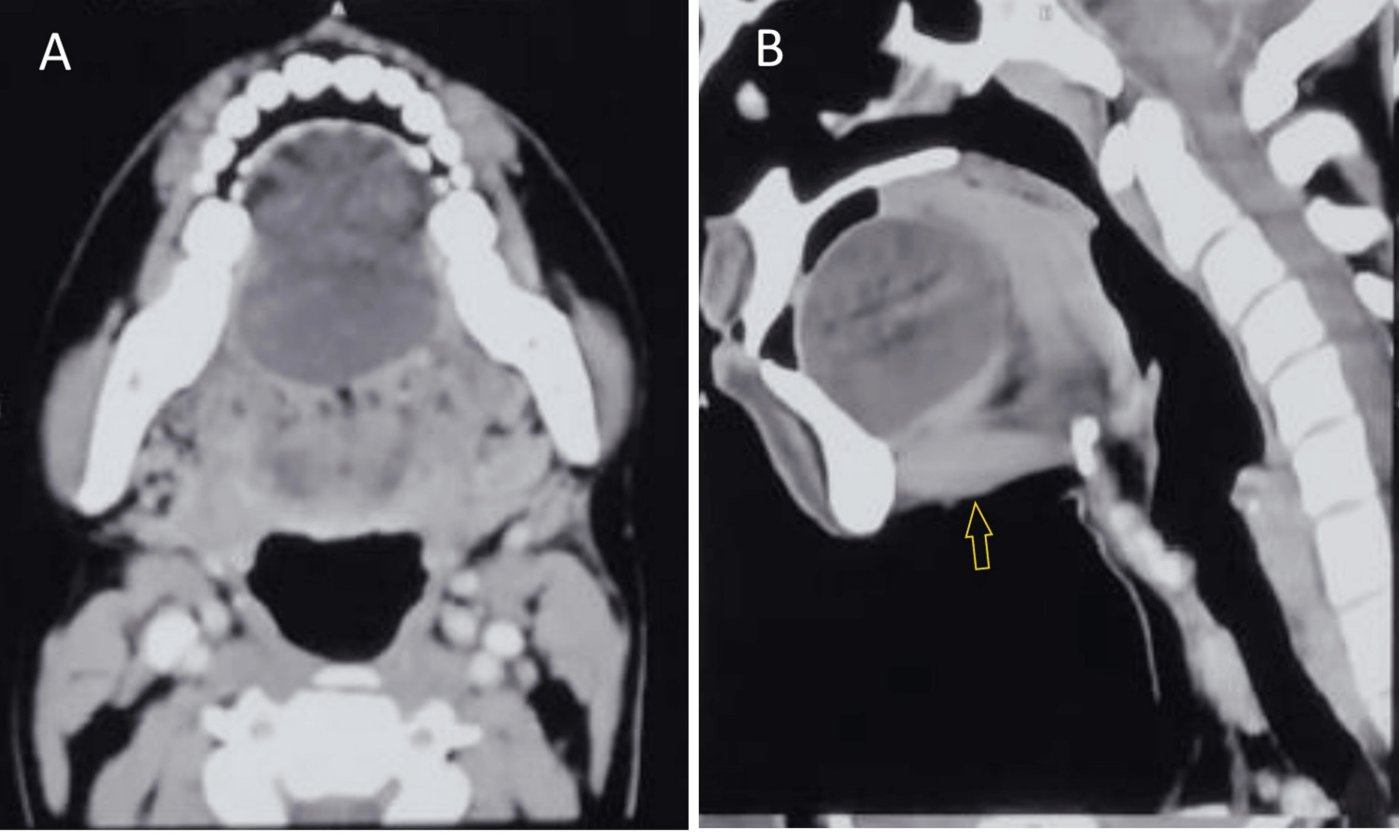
Axial (a) and sagittal (b) contrast-enhanced CT images showing a well-circumscribed cystic lesion in the sublingual space. The arrow denotes the mylohyoid muscle.
